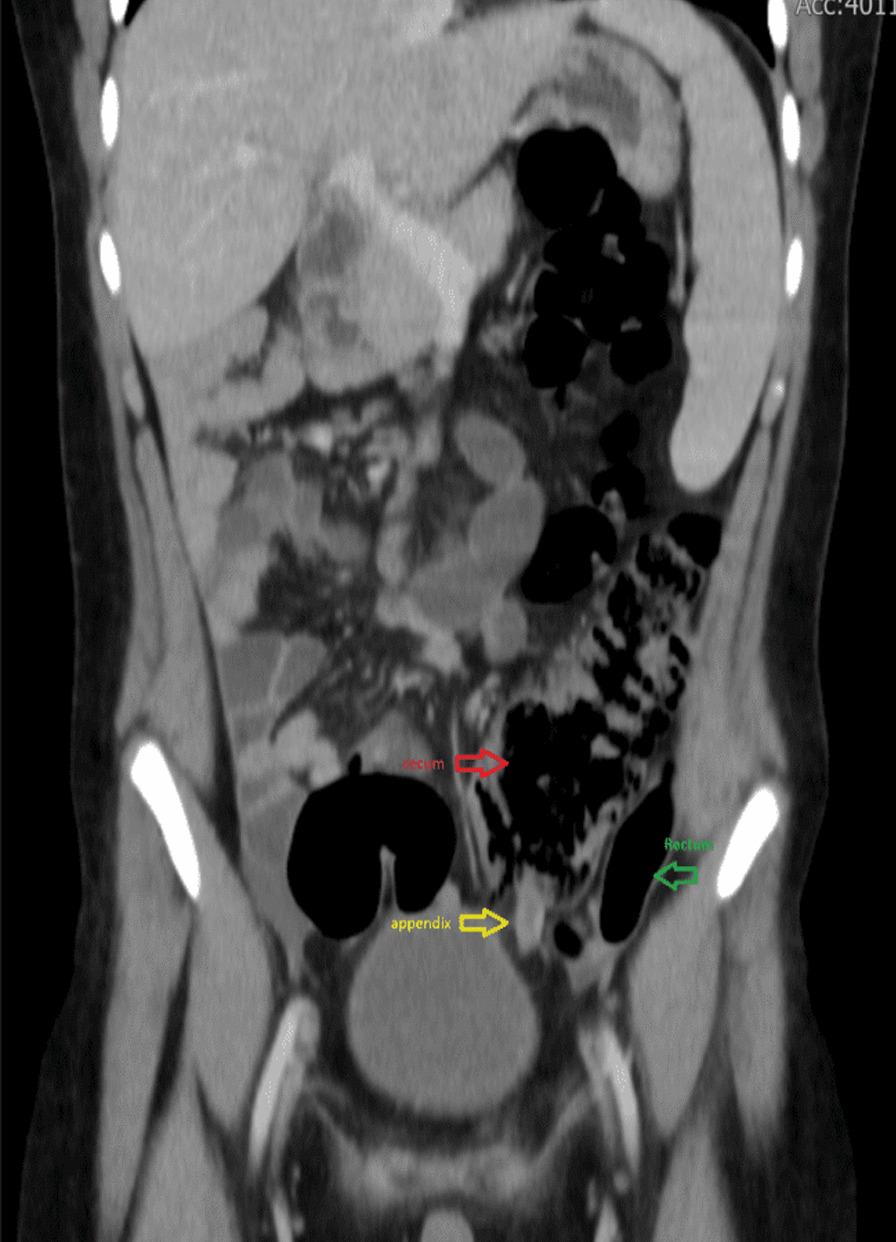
CT of the abdomen (coronal view) showing the appendix (yellow) between the cecum (red) and rectum (green)
